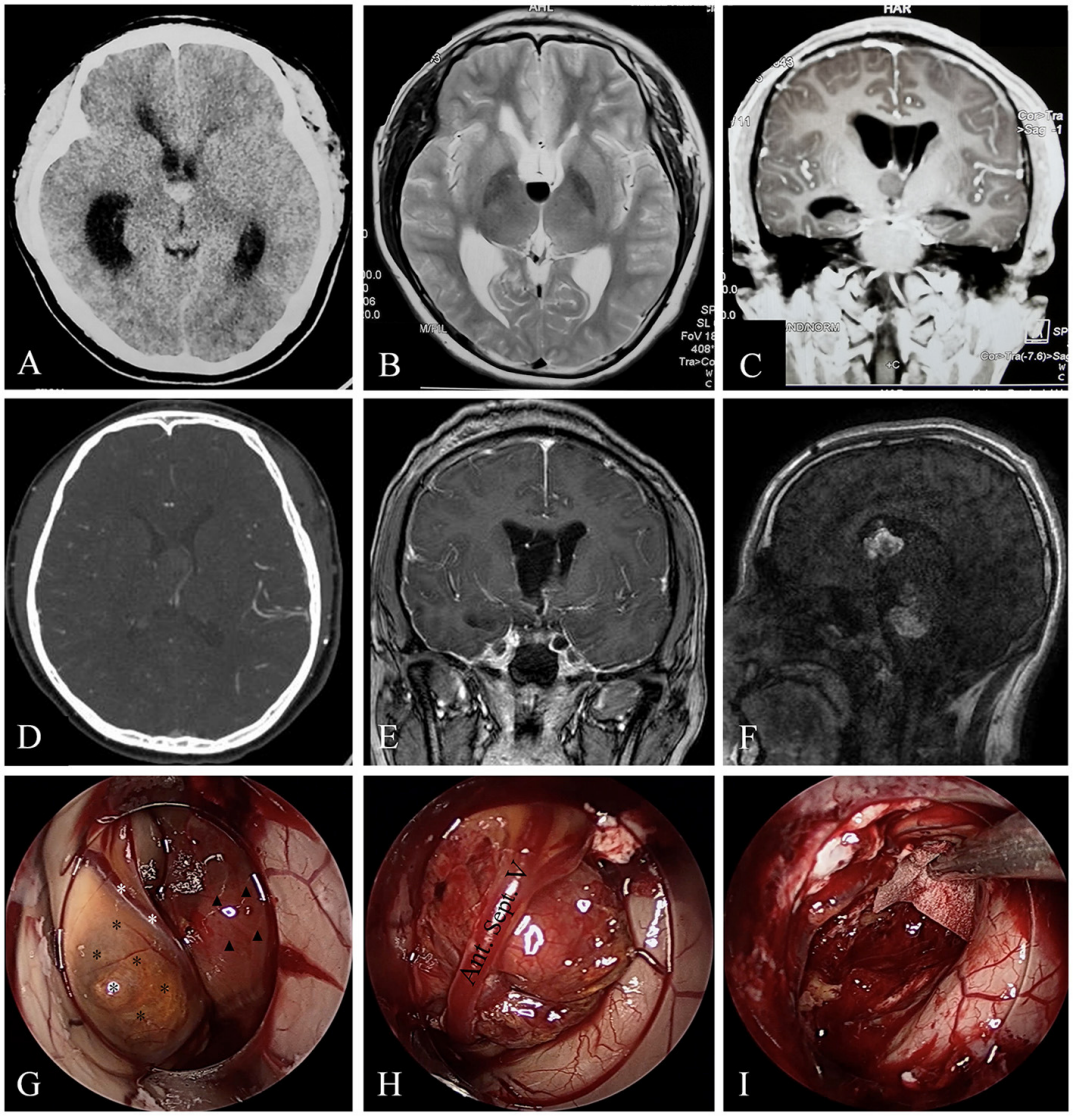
A rare case of CH originating from septum pellucidum with multiple recurrences. Case 9, the brain CT (A) of a 16-year-old boy revealed a hemorrhagic lesion of the third ventricle. T2-weighted image (B) showed fluid level in the lesion, suggesting hemorrhage. Coronal enhanced MRI (C) depicted a non-enhanced lesions causing obstructive hydrocephalus. Brain CTA (D) in our hospital revealed the lesion was without apparent blood supply. Endoscopic surgical resection was achieved in our hospital, and postoperative enhanced MRI showed gross total resection of the lesion (E). Gd-enhanced MRI (F) showed the two lesions were heterogeneously enhanced. Intraoperative images (G–I) revealed the lesion (black asterisks) originated from the septum pellucidum, compressing the anterior septum vein (white asterisks). A capsuled hematoma (triangles) could also be observed in the third ventricle (G). We carefully separated and protected the anterior septum vein (H) during the operation. At last, the lesion and hematoma were removed (I).
